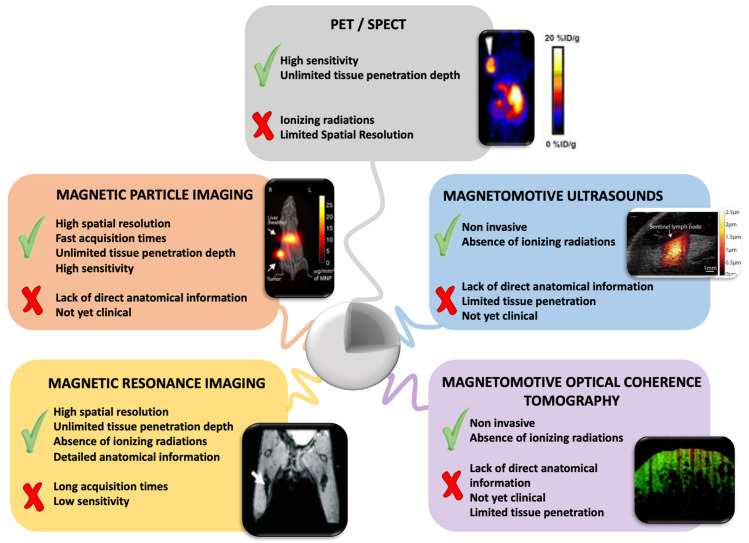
Advantages and limitations of the imaging techniques for tracking of MCs. MCs can be tracked by various imaging techniques (PET/SPECT; magnetic particle imaging, magnetomotive ultrasounds, magnetic resonance imaging, and magnetomotive optical coherence tomography). The selection of a precise technique is based on the assessment of the associated advantages and disadvantages. Adapted with permission from Refs. [364,365,366,367,368].
